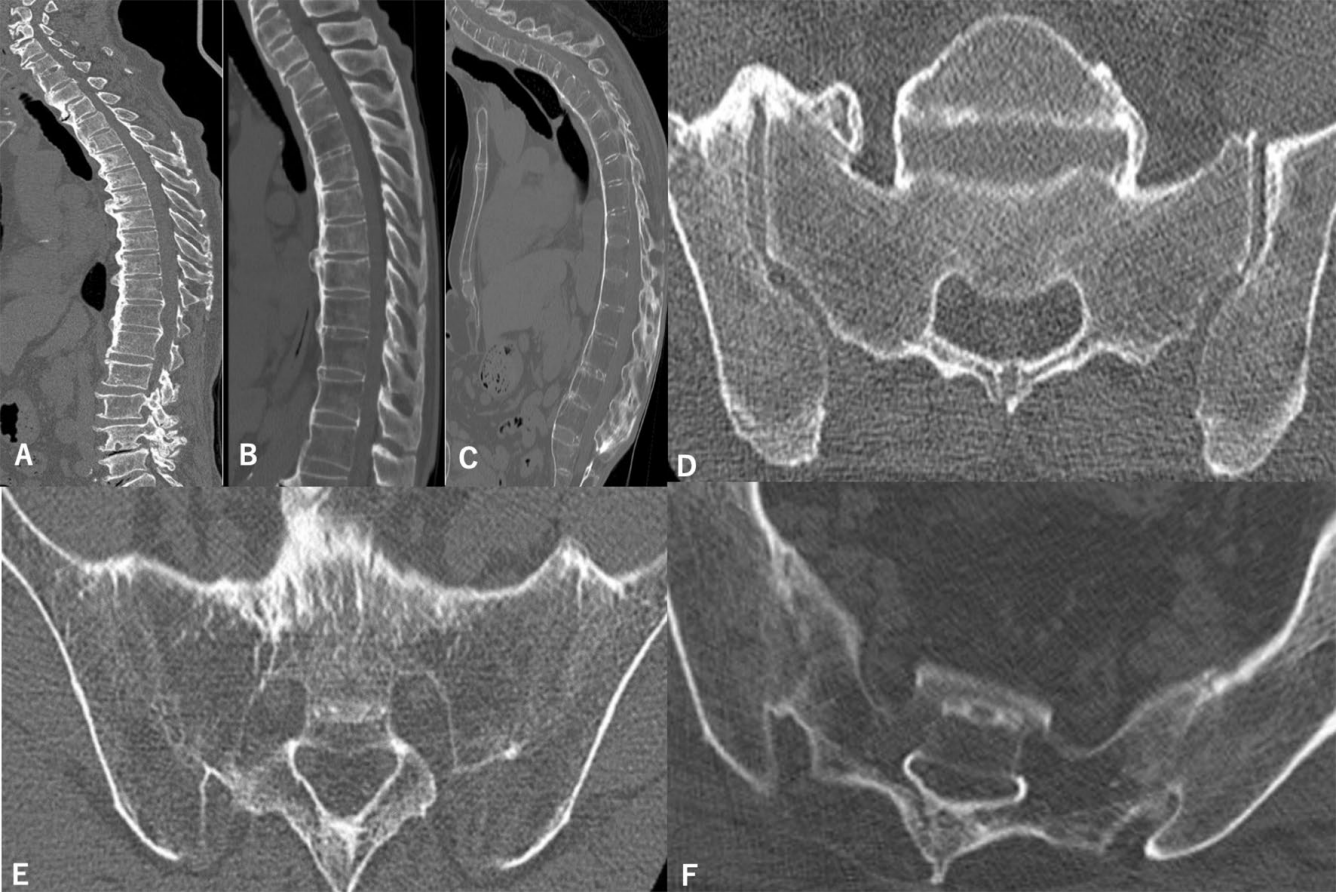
CT images of the case presentation. (A) Sagittal CT image of the whole spine shows 70–100% candle-wax-type in a 70-year-old male patient with DISH. (B) Sagittal CT image of the whole spine shows 0–30% candle-wax-type in an 82-year-old male patient with DISH. (C) Sagittal CT image of the whole spine shows 0–30% candle-wax-type in a 54-year-old male patient with AS. (D) Axial CT image of SIJ shows anterior bony bridging of the right side and no joint fusion in a 78-year-old male patient with DISH. (E) Axial CT image of the SIJ shows complete joint fusion in a 75-year-old male patient with DISH. (F) Axial CT image of the SIJ shows complete joint fusion in a 63-year-old male patient with AS.
